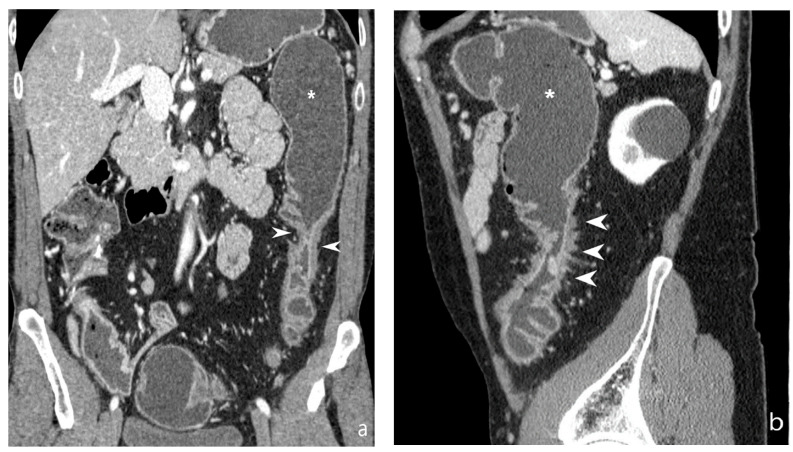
Intestinal stenosis: CT-E after iodinated contrast injection and administration of polyethylene glycol solution; coronal image (a) and sagittal image (b) show tight stenosis of the descendent colon (arrowheads), with stratified contrast enhancement; mild overdistention of the upstream descending colon (asterisk) is evident. Original figures by LMM and LL.
